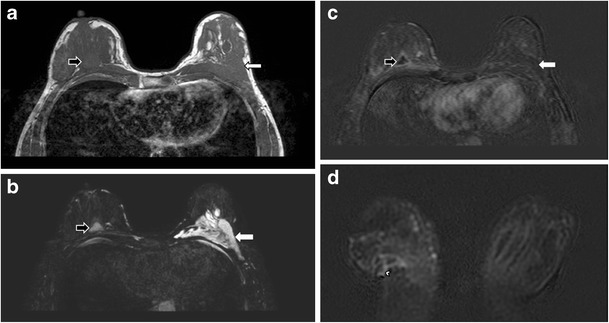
PAAG injection complicated with recurrent right breast abscess. Axial MR images of T1-weighted (a) and T2-weighted, fat-saturated (b) sequences depict a T1 hypointense and T2 hyperintense collection (black arrows) at the right breast. It shows rim enhancement after contrast injection (c). A sinus tract (arrowhead) is demonstrated at the lower outer aspect of the collection on the T1-weighted post-contrast coronal image with subtraction (d). Note the T1 low signal (a), T2 high signal (b) PAAG collection without any enhancement at the left retroglandular region (white arrows) (c)
